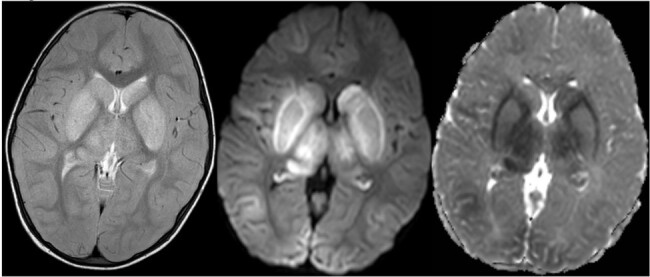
T2 TSE showing hyperintensities in bilateral basal ganglia (caudate nucleus, putamen and globus pallidus) and thalamus and corresponding DWI and ADC images showing true diffusion restriction
